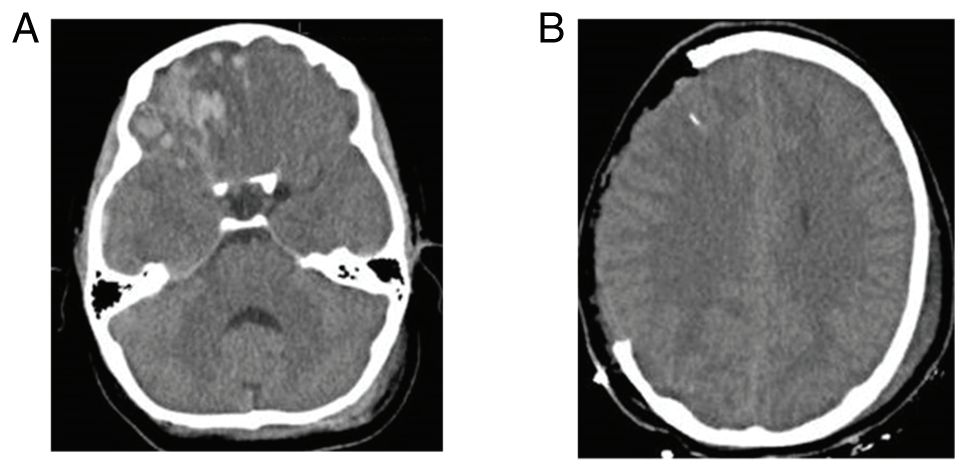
A 9-year-old female patient was admitted with a Glasgow Coma Scale score of 10 following severe traumatic brain injury and anisocoria (pupil right>left). (A) The first (pre-operative) computed tomography scan revealed the following: Brain contusions, edema and subdural hematoma, with an elevated intracranial pressure (>22 mmHg). (B) Post-operative computed tomography scan following decompressive craniectomy.
